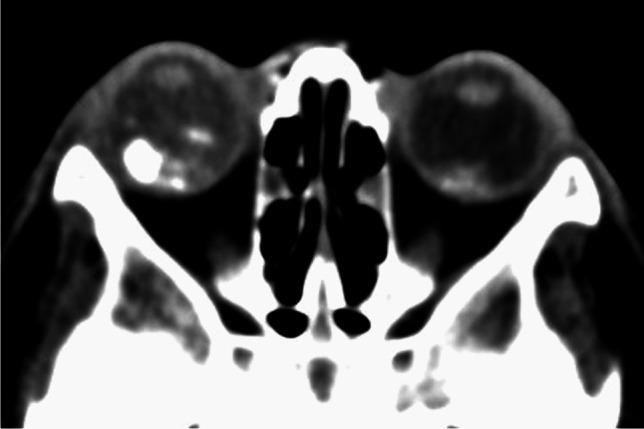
Bilateral retinoblastoma. There are right greater than left intraocular masses containing calcifications on this axial CT image of the orbits with soft tissue algorithm and window
